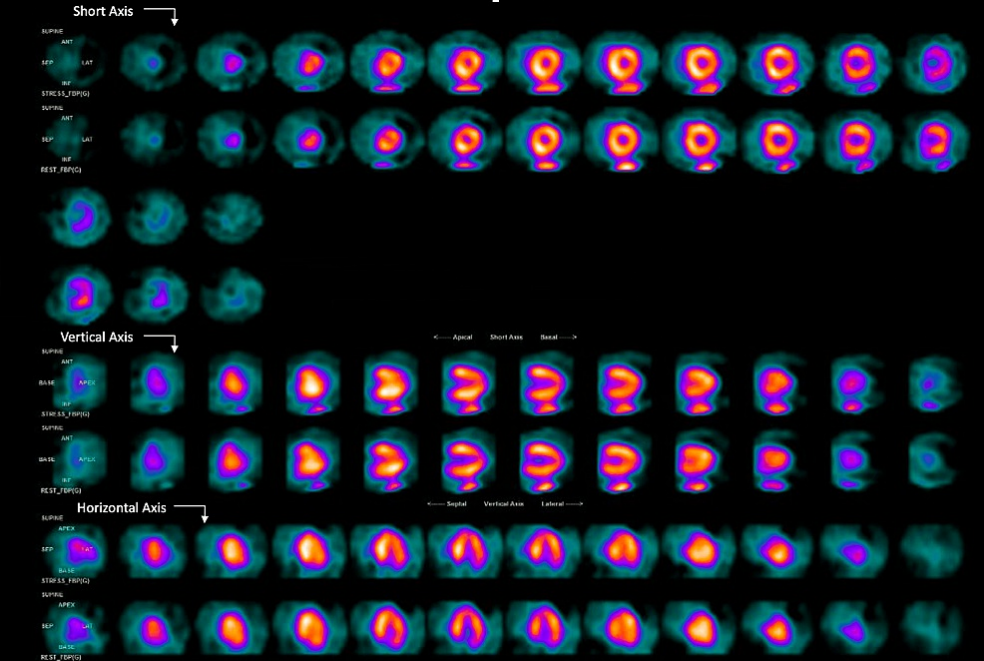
Myocardial perfusion imaging Non-ischemic SPECT (single-photon emission computed tomography) scan.
